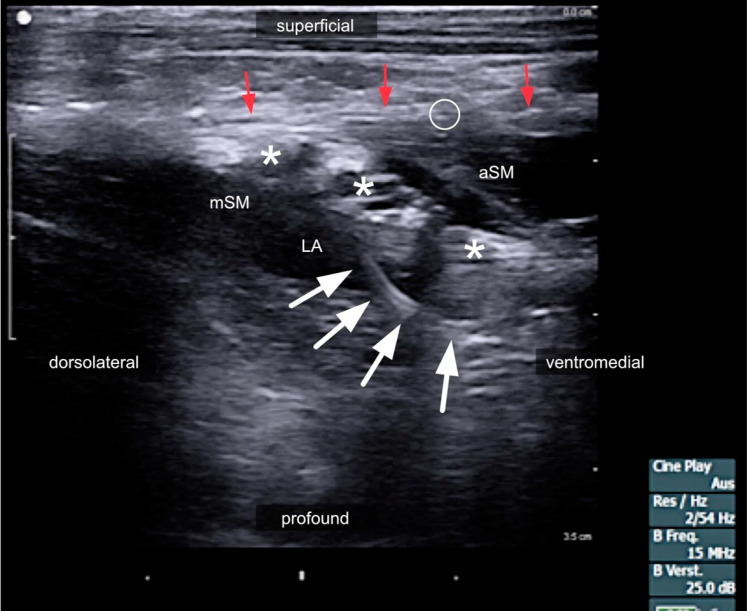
Sonogram of the left lateral neck region after the injection of 1 mL of local anesthetic via an interscalene catheter. White stars: brachial plexus; red arrows: prevertebral fascia; white arrowheads mark the catheter in situ; white circle: phrenic nerve; LA: local anesthetic; aSM: anterior scalene muscle; SCM sternocleidomastoid muscle; mSM: middle scalene muscle.
